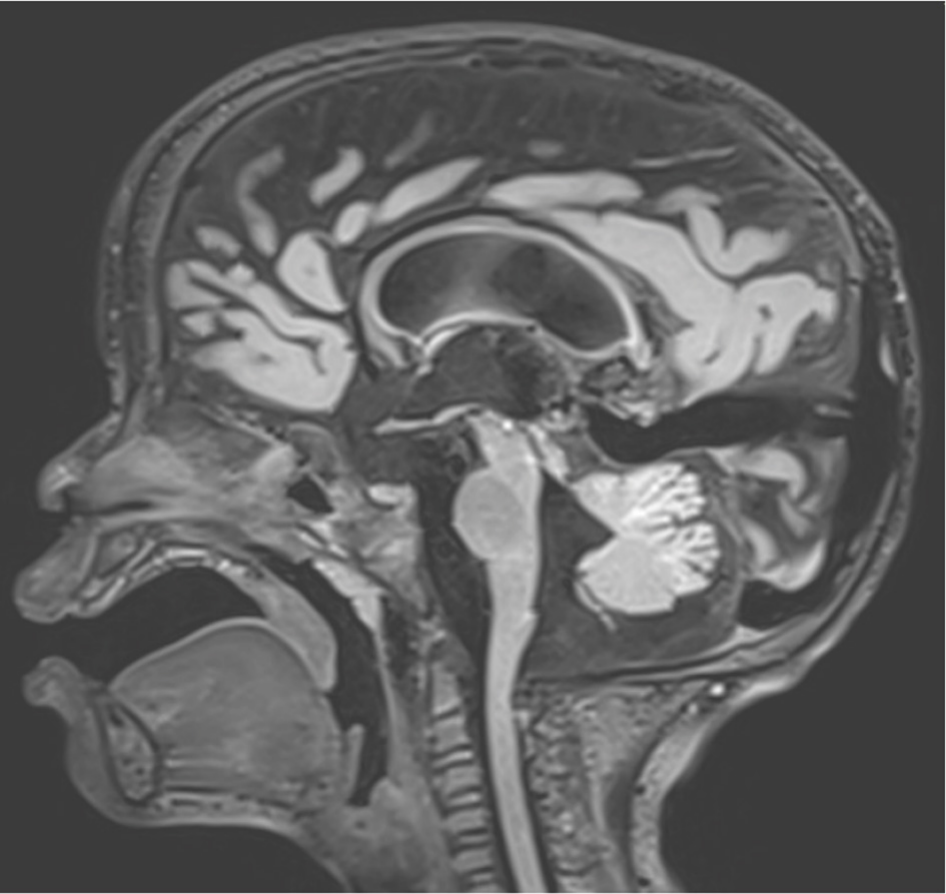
Sagittal FLAIR‑weighted MRI image showing cerebellar atrophy following opioid‑induced toxicity. A pre‑existing cystic malformation of the posterior fossa, consistent with a Blake’s pouch cyst, remains visible.
